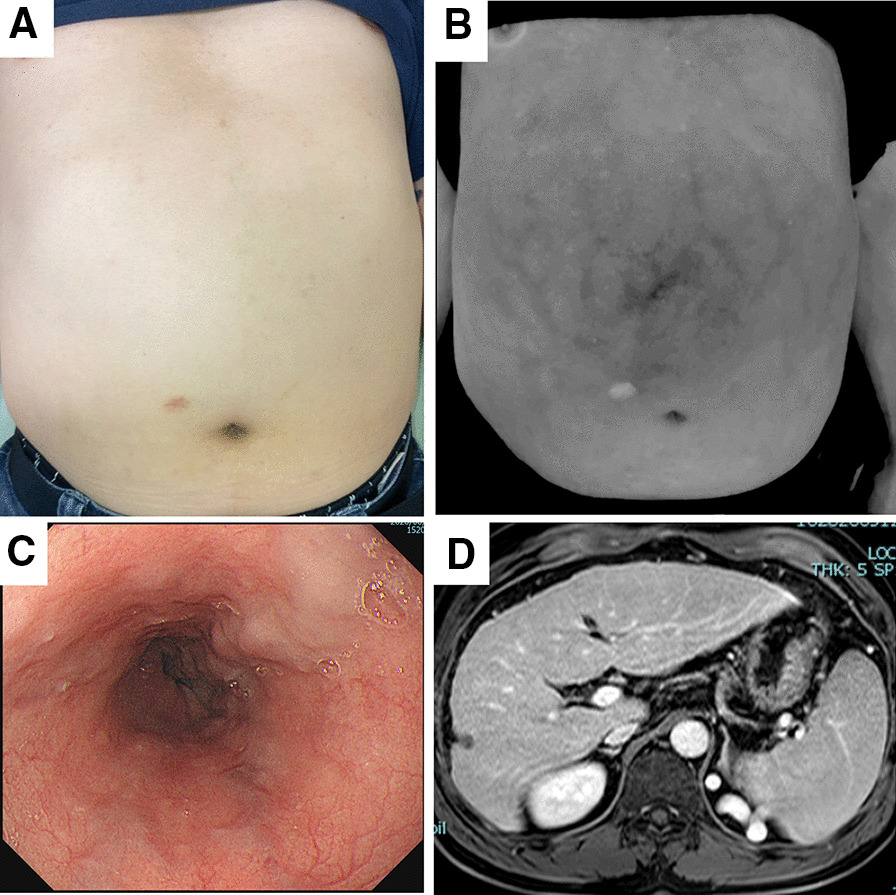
A representative case of VWI-AWV grade 2 whose UI-AWV was grade 0. a UI-AWV image classified grade 0, b VWI-AWV image classified grade 2, c Esophageal varices classified F2, d MRI showed cirrhosis and splenomegaly. *AWV* abdominal wall varices, *MRI* magnetic resonance of imaging, *UI* Unmanipulated image, *VWI* Vein-weighted image
